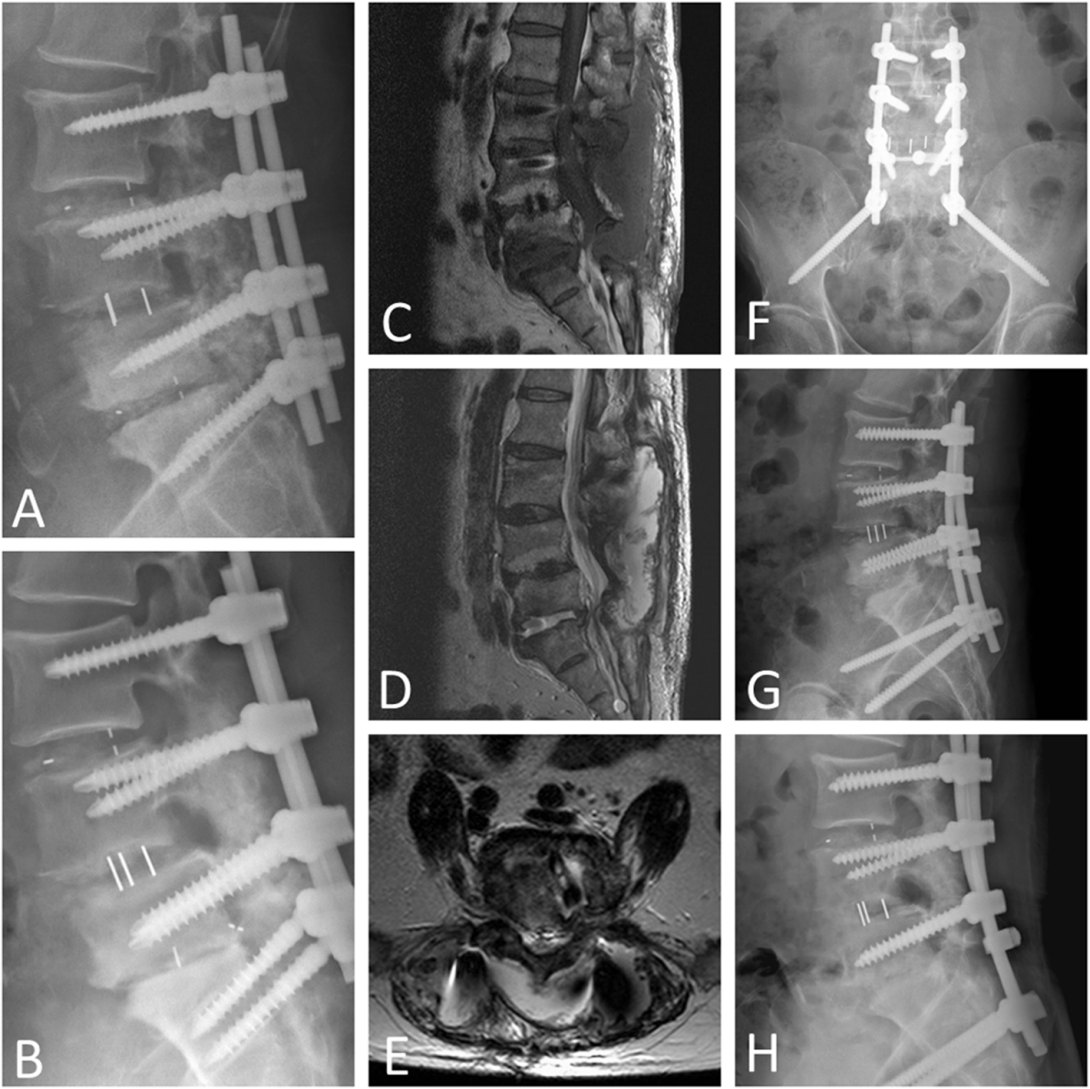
A A 57-year-old man underwent L3–S1 fusion with screws and cages. An initial postoperative X-ray showed good locations of the implants. B One month after the surgery, a follow-up X-ray showed posterior migration of the L5–S1 cage and suspected concurrent S1 screw loosening. C, D, E Magnetic resonance imaging revealed large amount of fluid accumulation in the L3–S1 subfascial, epidural, posterolateral regions, and L4–L5 disc space. F, G Posterior-alone surgery with extension of the distal instrumented level, removal of the L5–S1 cage, and L5–S1 TLIDF with allograft were performed. An initial postoperative X-ray showed good alignment. H One year later, an X-ray revealed L5–S1 solid interbody union, and the infection was well controlled.
